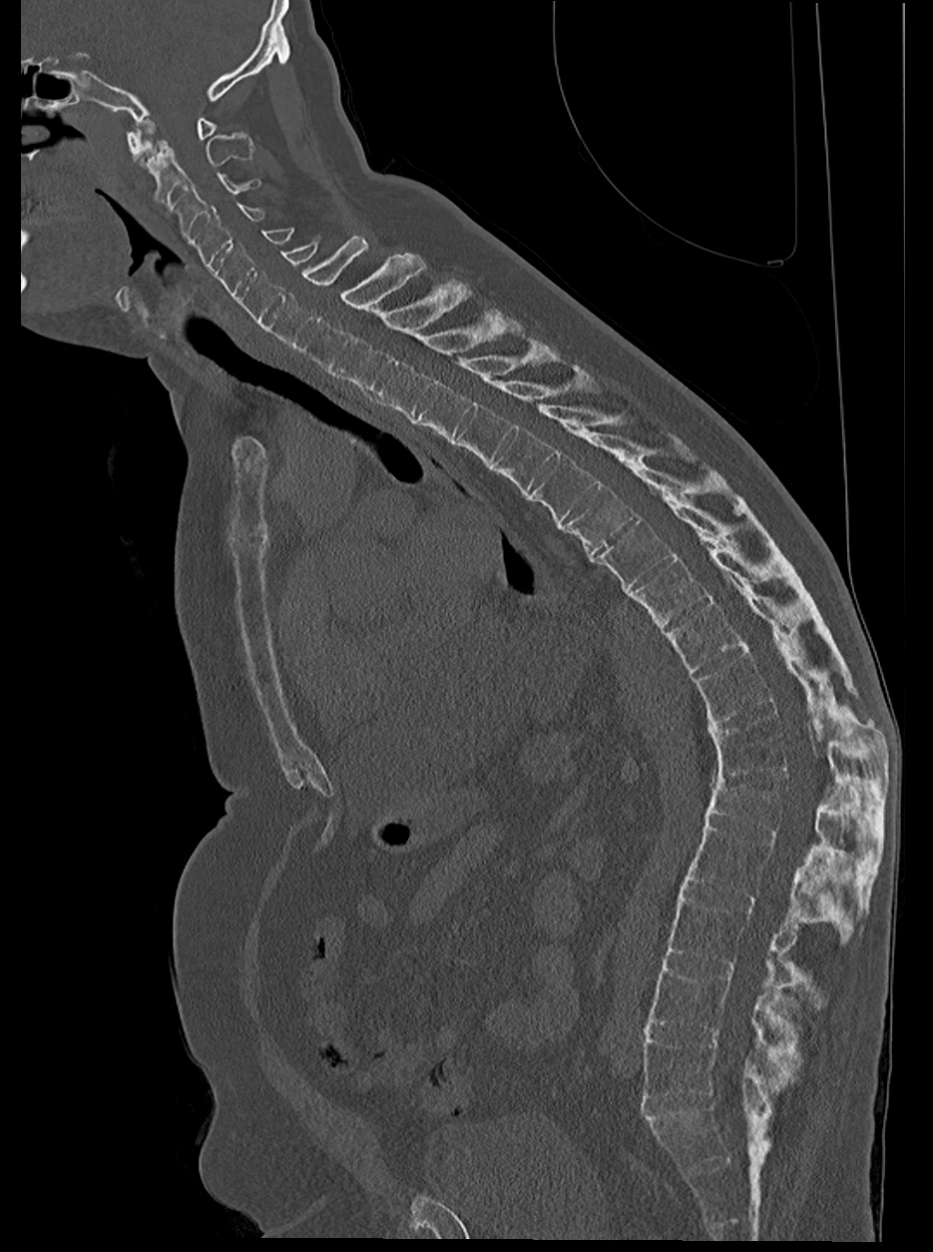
The CT scan with sagittal reconstruction shows the ossification and the global kyphosis of the whole spine and the old fracture at the level L1 healed in a kyphotic position.
